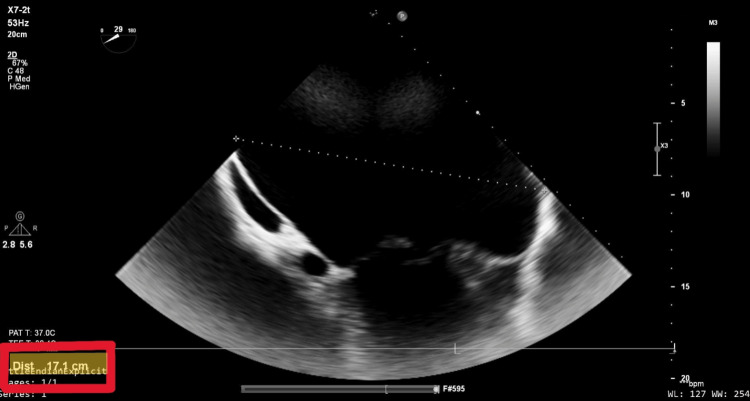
Transesophageal echocardiography Modified mid-esophageal view at 29°, selected to optimize visualization of the posterior left atrial wall. The anteroposterior diameter measures 17.1 cm, consistent with a giant left atrium. Adjacent cardiac structures are displaced, and typical chamber visualization is obscured due to the severe atrial enlargement.
